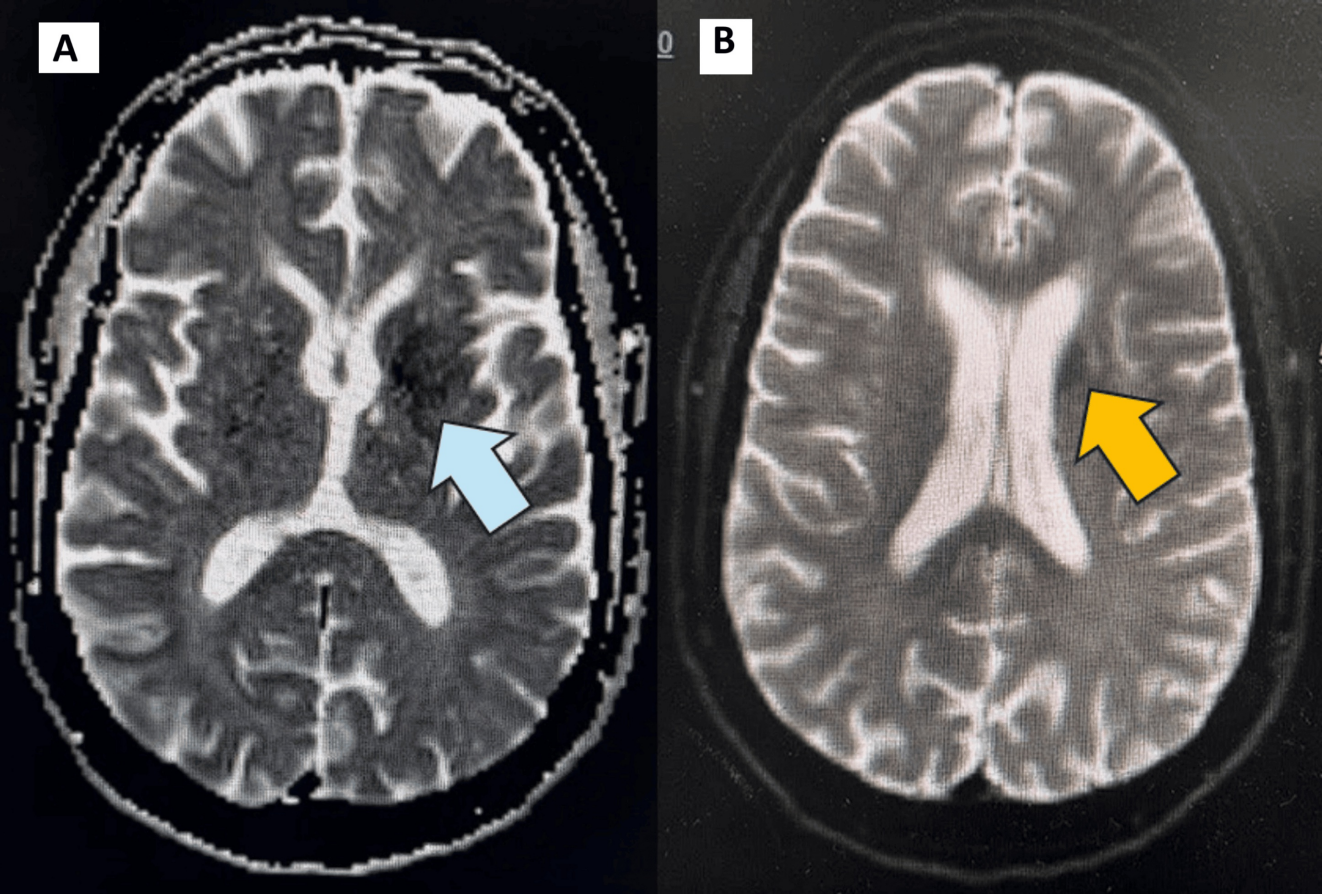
Brain MRI ADC and DWI Trace Sequences (A): Brain MRI ADC sequence. Blue arrow pointing at the left basal ganglia signal. (B): Brain MRI DWI trace sequence. Yellow arrow pointing at the left basal ganglia signal. ADC: Apparent diffusion coefficient; DWI: diffusion-weighted imaging
